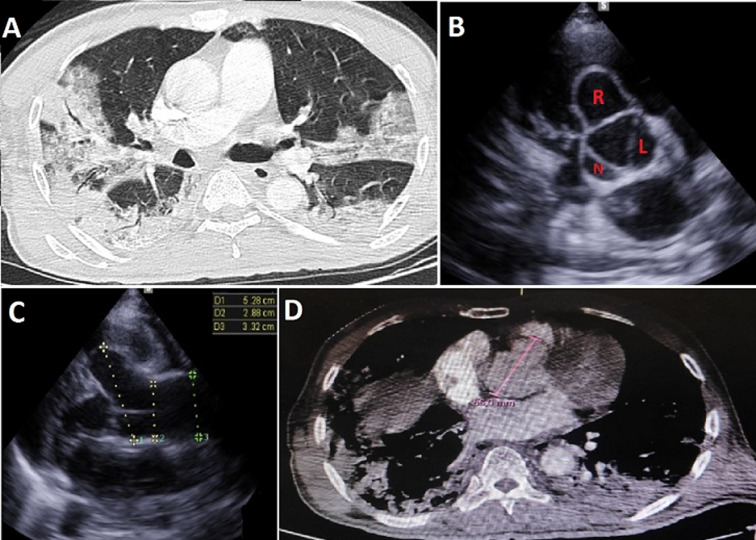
A) thoracic computed tomography scan-axial view showing diffuse patchy ground-glass like opacities suggesting COVID-19 pneumonia with severe involvement (75%); B) transthoracic echocardiogram - small axis parasternal view showing a dilated right sinus of valsalva (R: right sinus, L: left sinus, N: non coronary sinus); C) transthoracic echocardiogram - large axis parasternal view showing a dilated aortic sinus at 52.8 mm; D) thoracic computed tomography scan-axial view showing an aneurysm of right sinus of valsalva with diameter of aortic sinus at 56 mm
